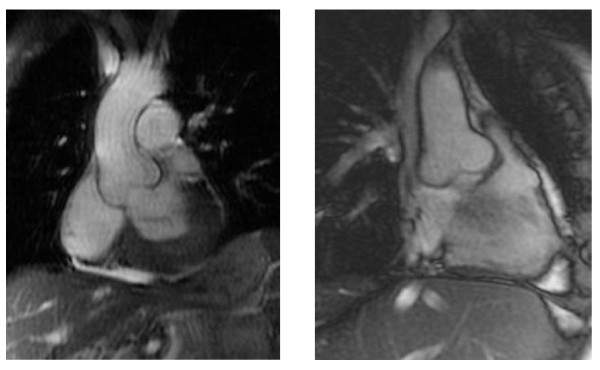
Aortic root dilatation in a patient suffering from Fabry disease: magnetic resonance imaging (MRI) showing aortic root dilatation in Fabry disease. Pr Dominique GERMAIN, University of Versailles - St Quentin en Yvelines (UVSQ), Versailles, France
